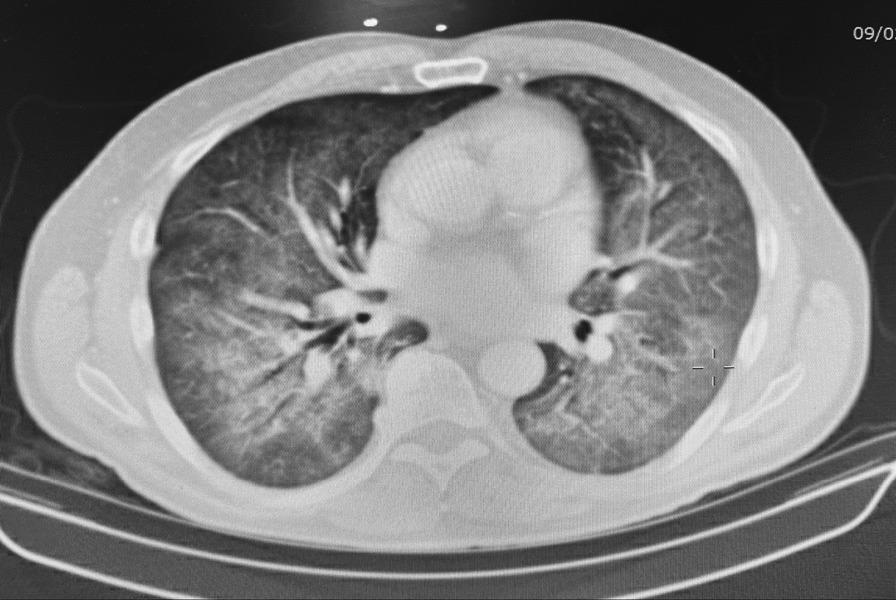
High resolution CT Chest with intravenous contrast. Notable for bilateral, patchy, and diffuse ground glass opacities in a batwing configuration
